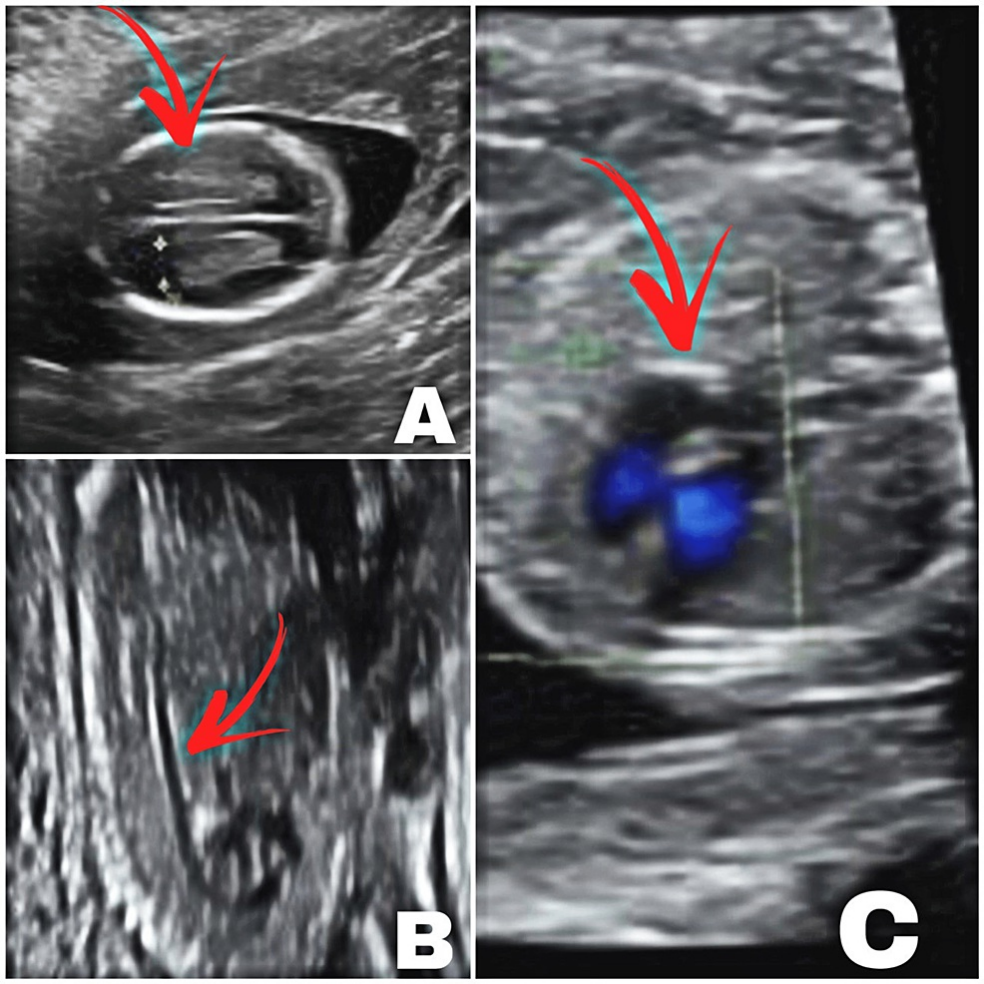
Ultrasound at 18 weeks revealed normal ventricular of the fetal head (A), with no structural or congenital anomalies in the spine (B). Four-chamber view of the heart (C)
